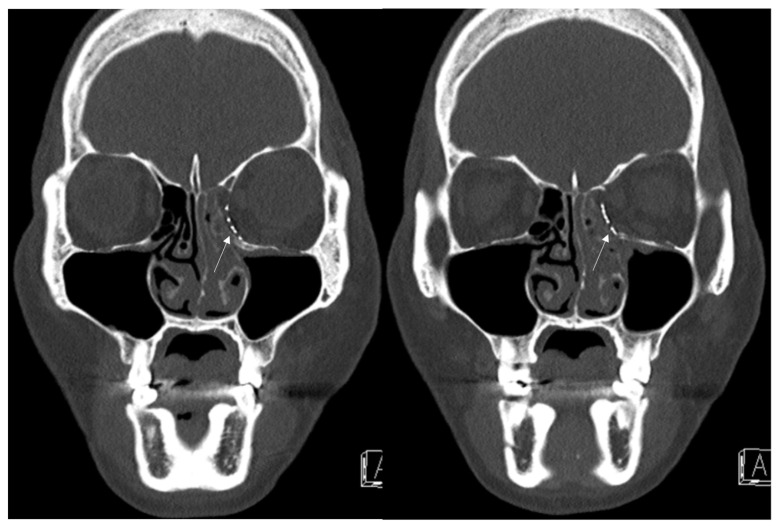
Case 2. Follow up facial CT finding (coronal view) of the same patient. The titanium-reinforced porous polyethylene (TR-PPE) plate was placed on the inferomedial orbital wall using a single screw at the inferior orbital rim. (White arrow).
